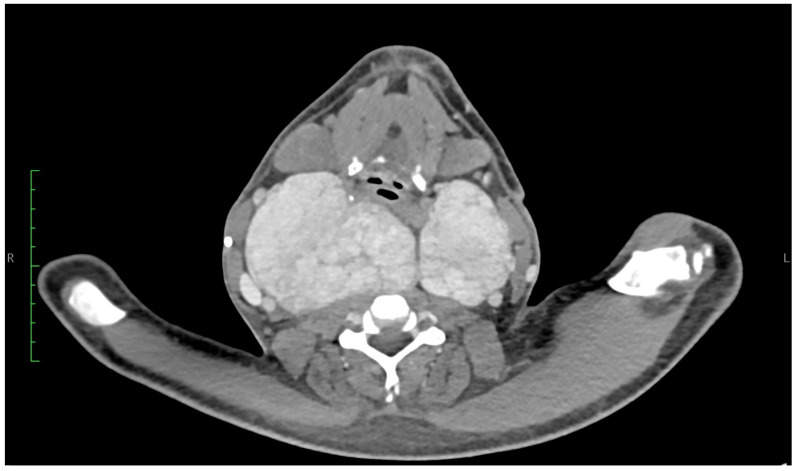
Neck CT. Frontal. The right thyroid lobe measures 74 × 89 × 140 mm (anteroposterior × right–left × craniocaudal). The upper pole reaches the level of the C1 vertebra, crosses the midline, and extends to the left side, displacing the larynx anteriorly and compressing the posterior right pharyngeal wall, resulting in narrowing of the airway. The left lobe measures 57 × 65 × 113 mm, with its upper pole reaching the level of the C2/C3 vertebrae. The lower poles of both lobes extend down to the superior border of the manubrium of the sternum. The isthmus measures up to 35 mm in width.
